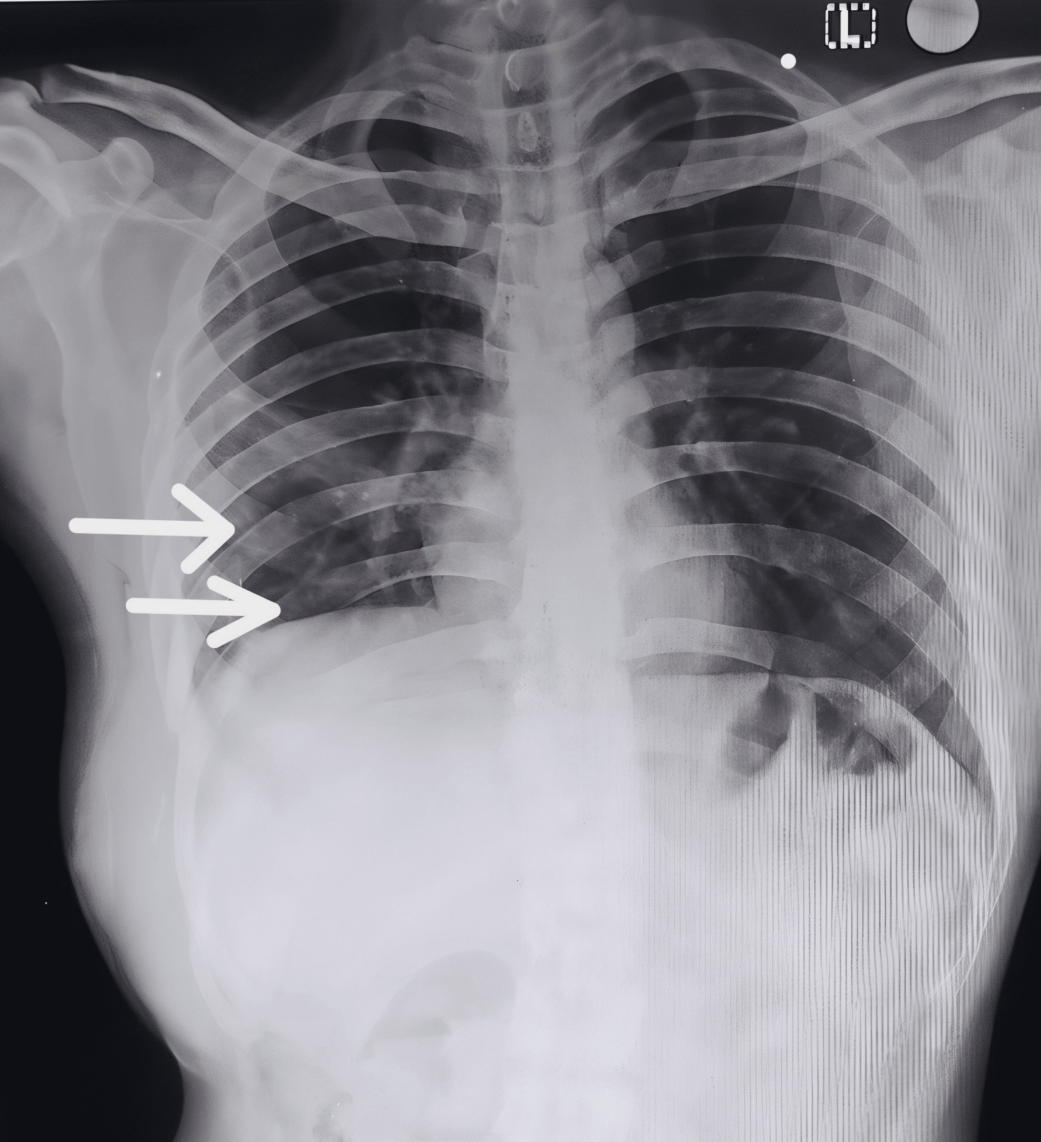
Chest X-ray of a 30-year-old male with suspected local invasion of malignant melanoma The posteroanterior chest X-ray of a 30-year-old male reveals lytic lesions in the eighth and ninth ribs on the right side, indicative of potential local invasion by malignant melanoma (white arrows).
